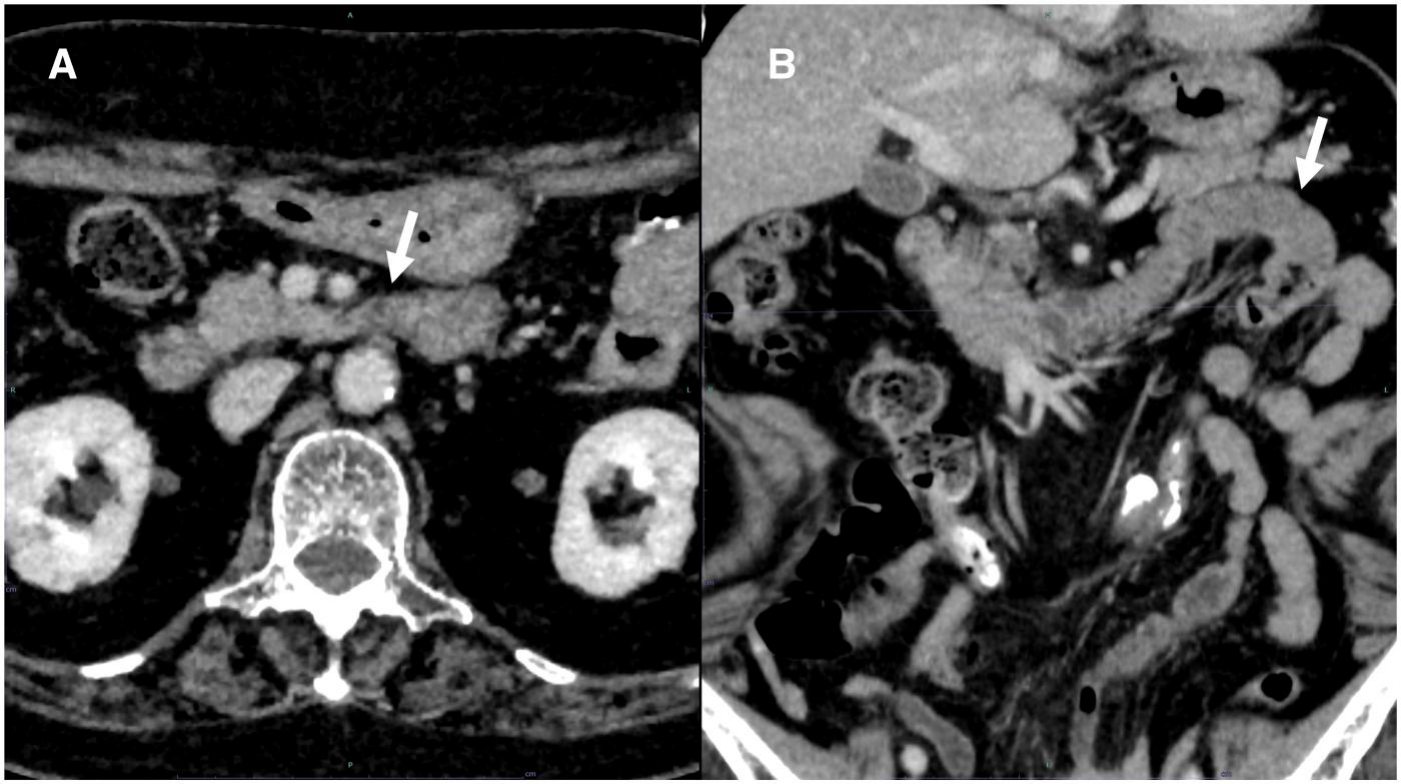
Contrast-enhanced CT obtained 7 days after the initial CT. The third part of the duodenum passes anterior to the aorta (A, arrow), and the jejunum is located on the patient’s left side (B, arrow), indicating spontaneous resolution of the previously right-sided duodenum and jejunum.
